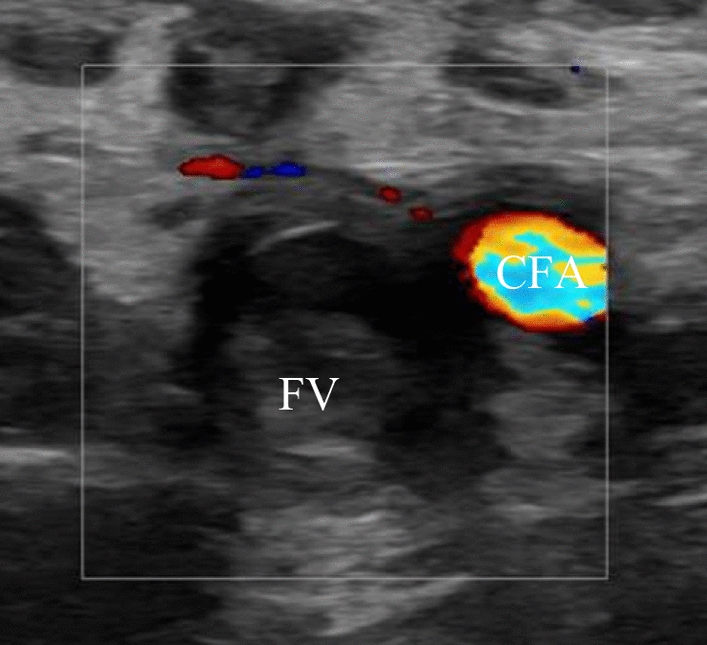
Doppler ultrasound showing no blood flow in the left femoral vein
